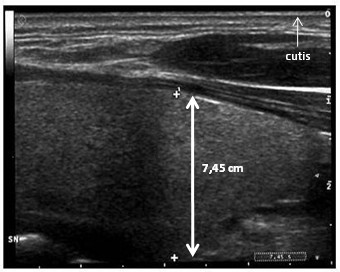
Ultrasonography scan of the cervical region. The scan evidenced a massive lipohypertrophy of the dorso-cervical region of the neck (the maximum diameter identified by focusing up and down through the planes of the section was 7.45 cm). The area of fat accumulation was extended over the entire cervical region.
